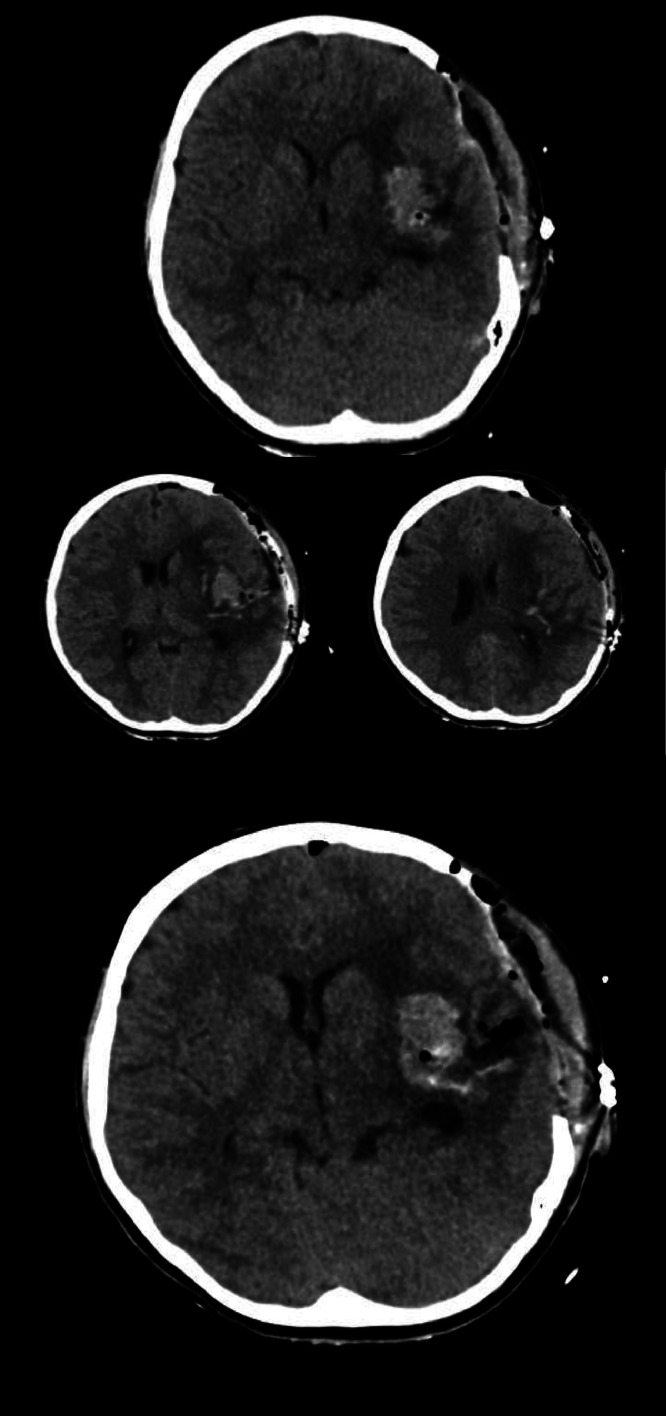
The first non-contrast CT brain following the postcraniotomy procedure. The non-contrast CT brain shows a large left parietal intraparenchymal hemorrhage with surrounding vasogenic edema, causing significant mass effect and a leftward midline shift. After the procedure, pneumocephalus is present. Further imaging, such as contrast-enhanced CT or vascular studies, may be required to assess for underlying vascular pathology.
